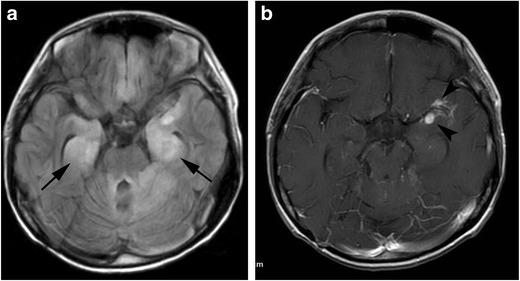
Paraneoplastic limbic encephalitis. A 17-year-old boy with recurrent medulloblastoma presented with seizure and altered mental status. a FLAIR image shows hyperintensities in the mesial temporal regions (*arrows*). b Contrast enhanced fat-suppressed T1-weighted image shows nodular meningeal enhancements in the left Sylvian fissure (*arrowheads*), suggestive of recurrence
